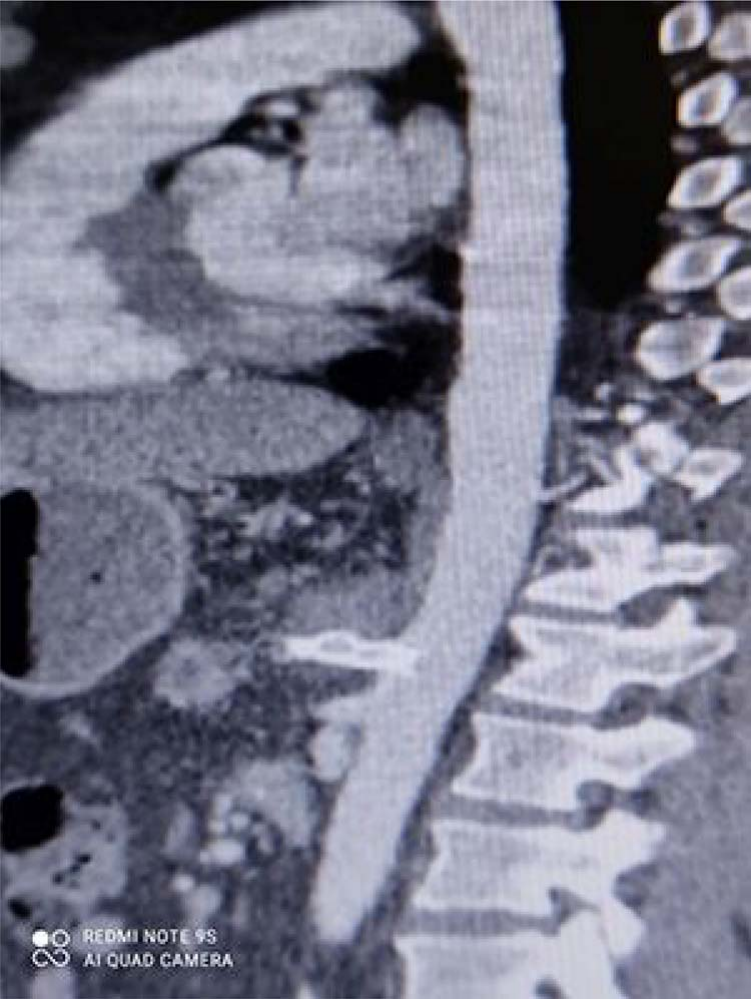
CT angiography showing repaired CAA with no leakage.
